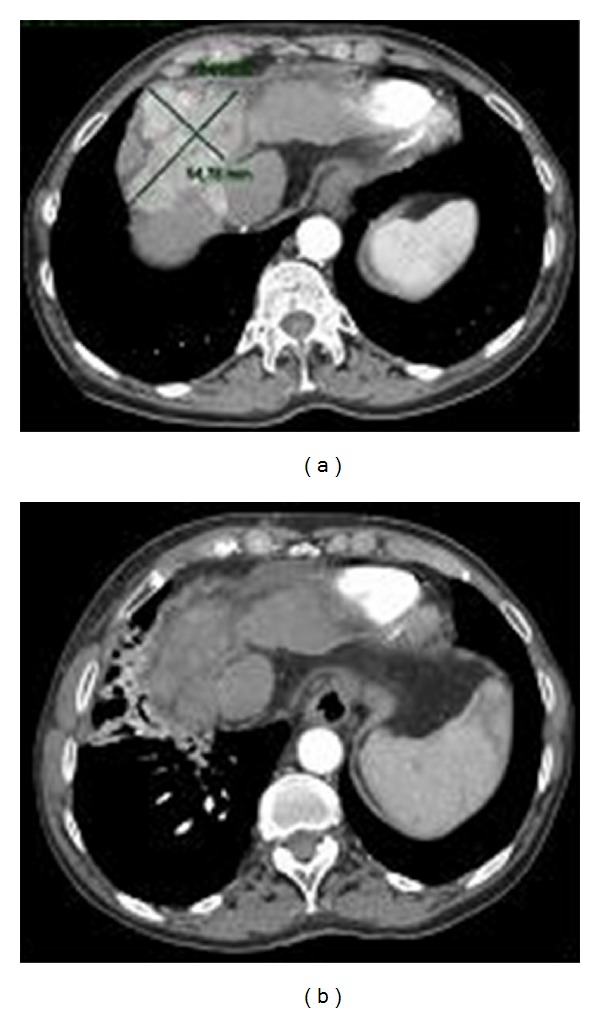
Abdominal computed tomography scans before and 6 months after HSRT show a complete disappearance of hypervascular arterial enhancement of primary tumour.
